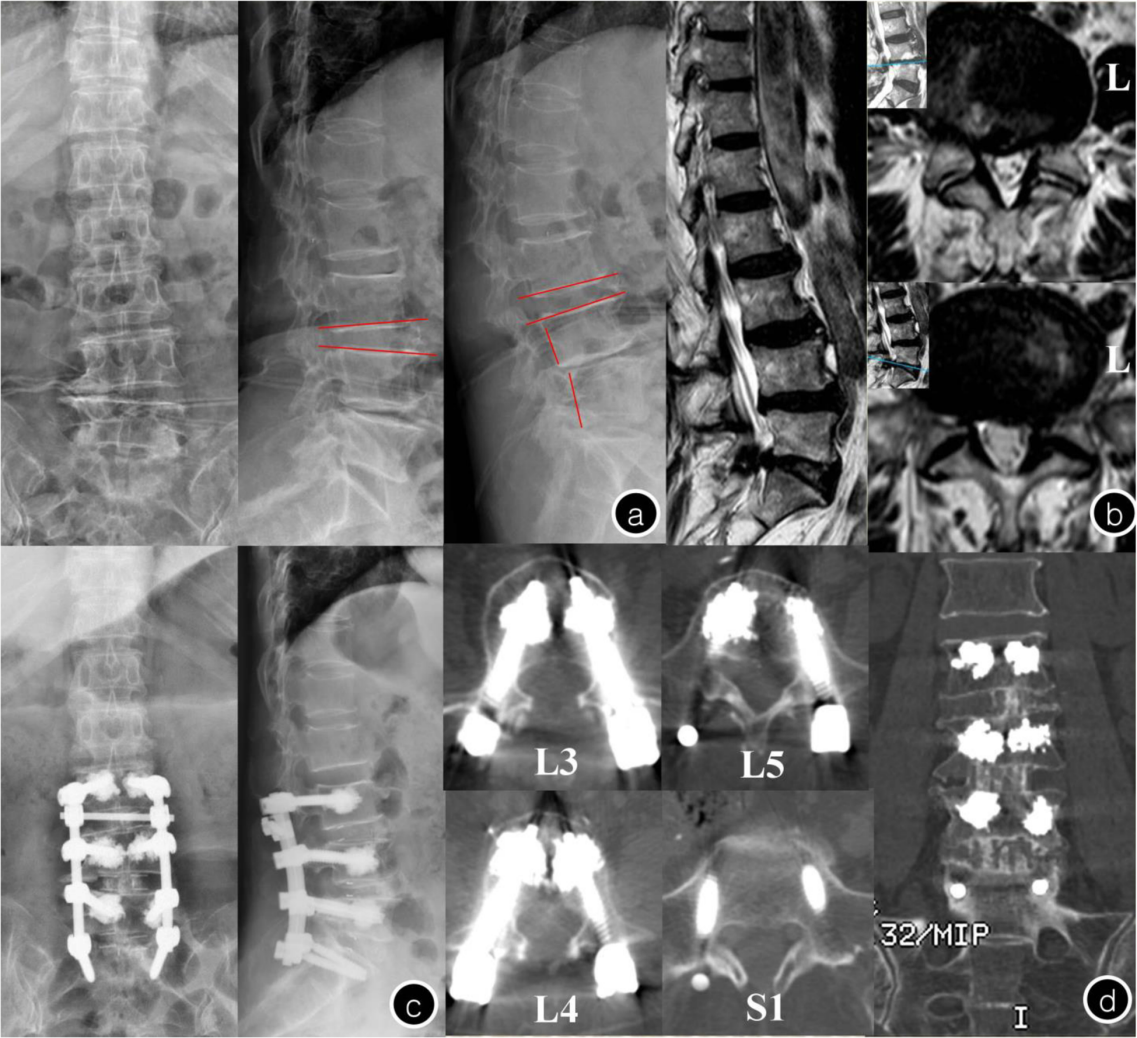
Female, 75-year-old, T = -3.8 SD a. Preoperative x-ray image showed lumbar vertebral degeneration with spinal instability in L3-L5; b. Preoperative MRI: L4/5 spinal canal stenosis and L5-S1 level lumbar disk herniation; c. Postoperative x-ray: the internal fixation was in good position and without serious leakage of cement; d. Five years after the operation, the CT showed that pedicle screw has not loosened and the L3-S1 was interbody fusion
